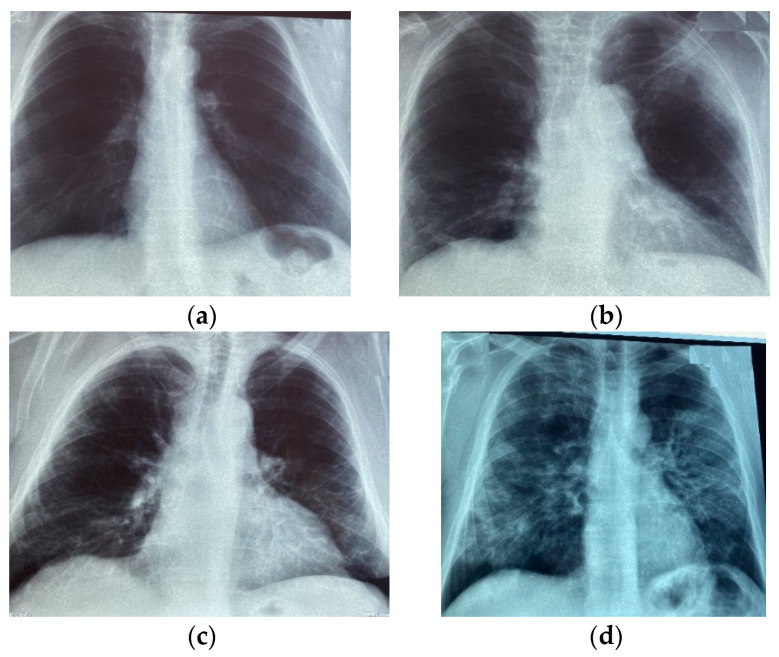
Chest X-ray images labelled with different COVID-19 severity score: (a) Normal; (b) Mild; (c) Moderate; (d) Severe.
